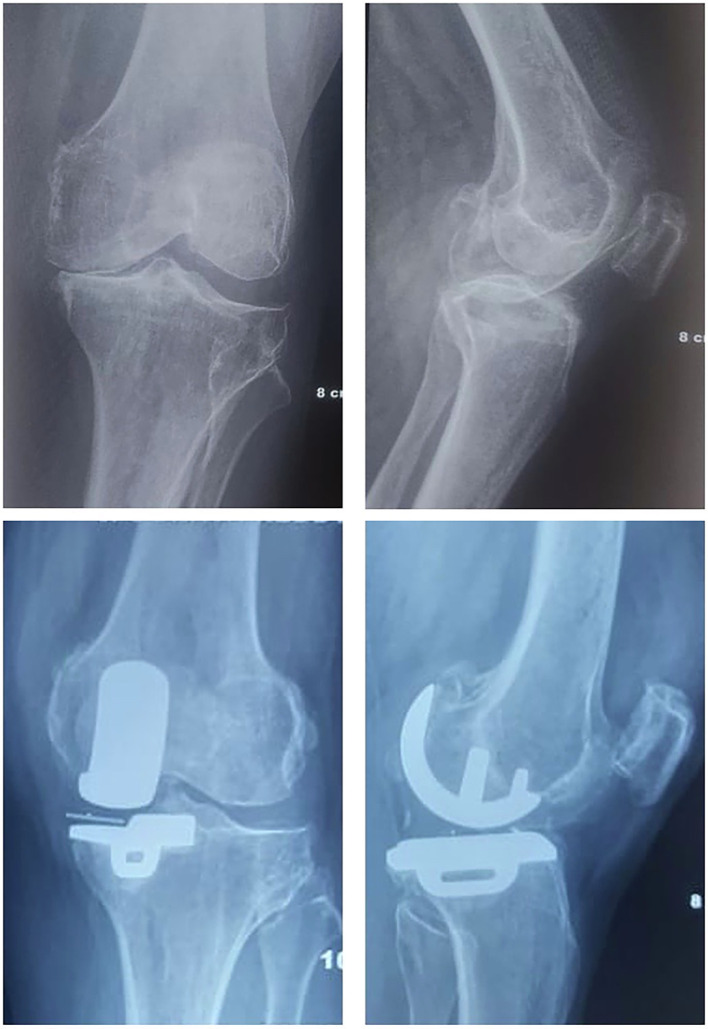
Pre- and Post-operative radiographs of Oxford mobile bearing UKA for medial OA of the knee.
